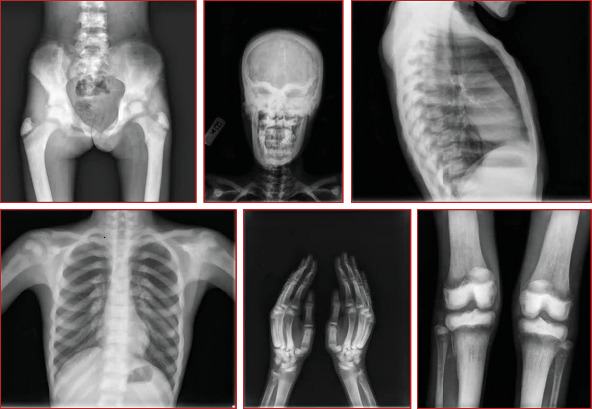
Limb radiographs show increased bone density and increased cortical thickness. Skull X-ray shows lytic lesions and skull base sclerosis.
